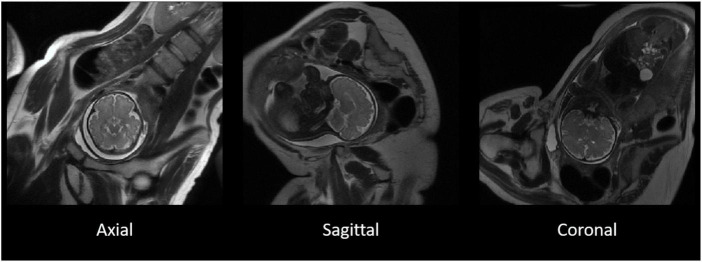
The original T2-weighted acquisition of a fetal MR image in axial, sagittal, and coronal planes. T2-weighted images acquired separately in three separate image planes in the axial (left), sagittal (middle), and coronal (right) in a representative participant. The three image planes were subsequently used for the reconstruction of 3D images.
